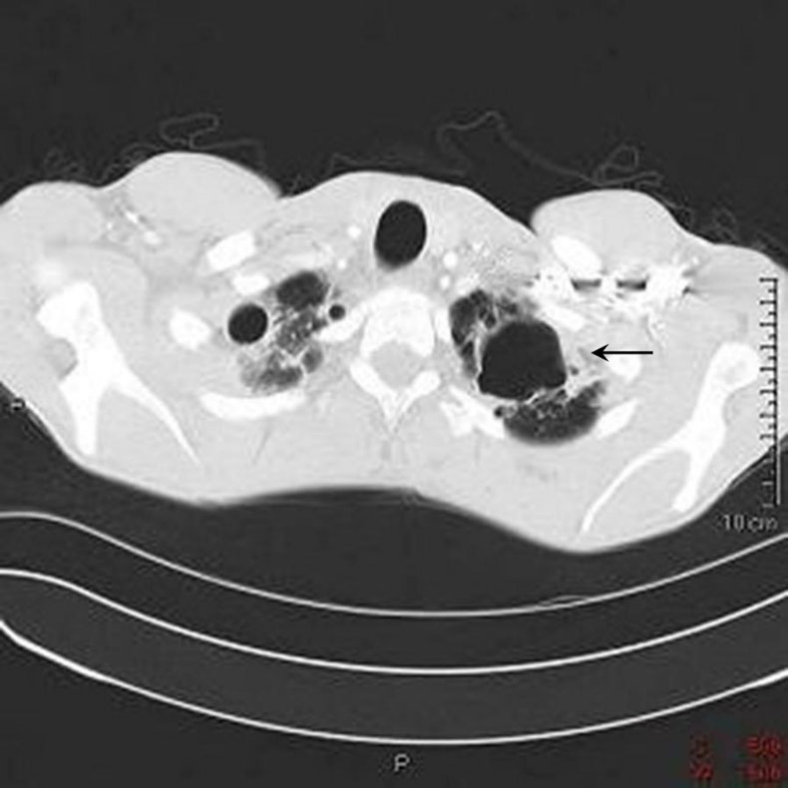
High resolution CT scan of the patient's lungs demonstrating apical bullae. Note the prominent bronchial arteries (arrow).
